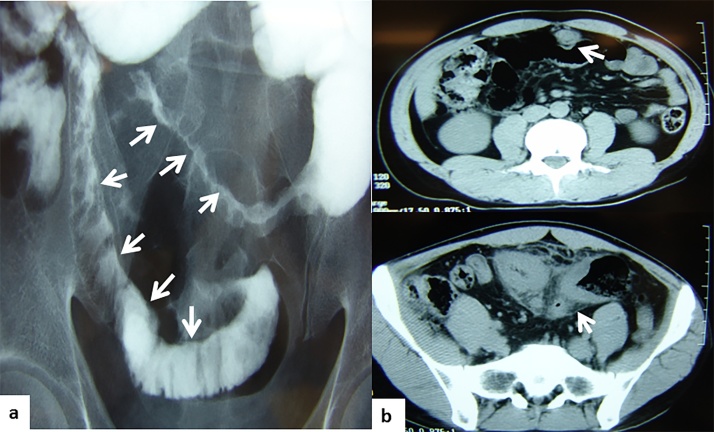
a) A longitudinal ulcer is noted in a small bowel series. b) urachal mass extends into the umbilicus on computed tomography.
